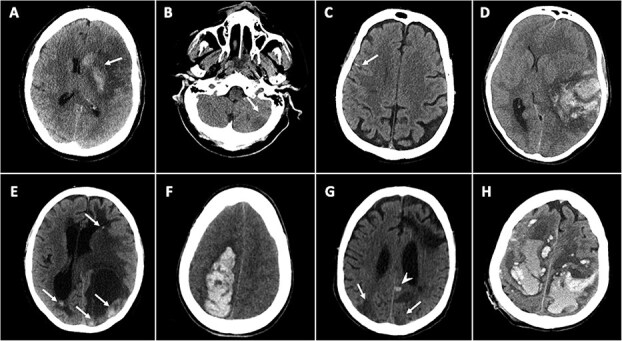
Examples of non-haemorrhagic NCCT findings suggestive of secondary causes of ICH. (A) Left lenticulo-striatal haemorrhage (arrow) in the context of an ischaemic stroke in the superior middle cerebral artery territory, suggestive of haemorrhagic transformation. (B) Small hypodensity in the left cerebellar hemisphere (B) in a non-arterial vascular territory, suggestive of venous infarction. (C) Multiple linear hyperdensities in frontal sulci indicating cortical venous thrombosis. (D) Irregular and heterogeneous haemorrhage with absence of small vessel disease CT markers (no leukoaraiosis or lacunes). (E) Multiple haemorrhages with surrounding oedema secondary to embolic strokes. (F) Right parieto-frontal haemorrhage with absence of adjacent of CT features typically associated with sporadic cerebral amyloid angiopathy (ie, finger-like projections and focal subarachnoid haemorrhages). (G) Multiple, concomitant hypodense and hyperdense lesions (arrows) in a patient with a PRES. (H) Multiple haemorrhages in a patient with acute leukemia, “polka dot sign”. Abbreviations: NCCT = non-contrast CT; PRES = posterior reversible encephalopathy syndrome.
